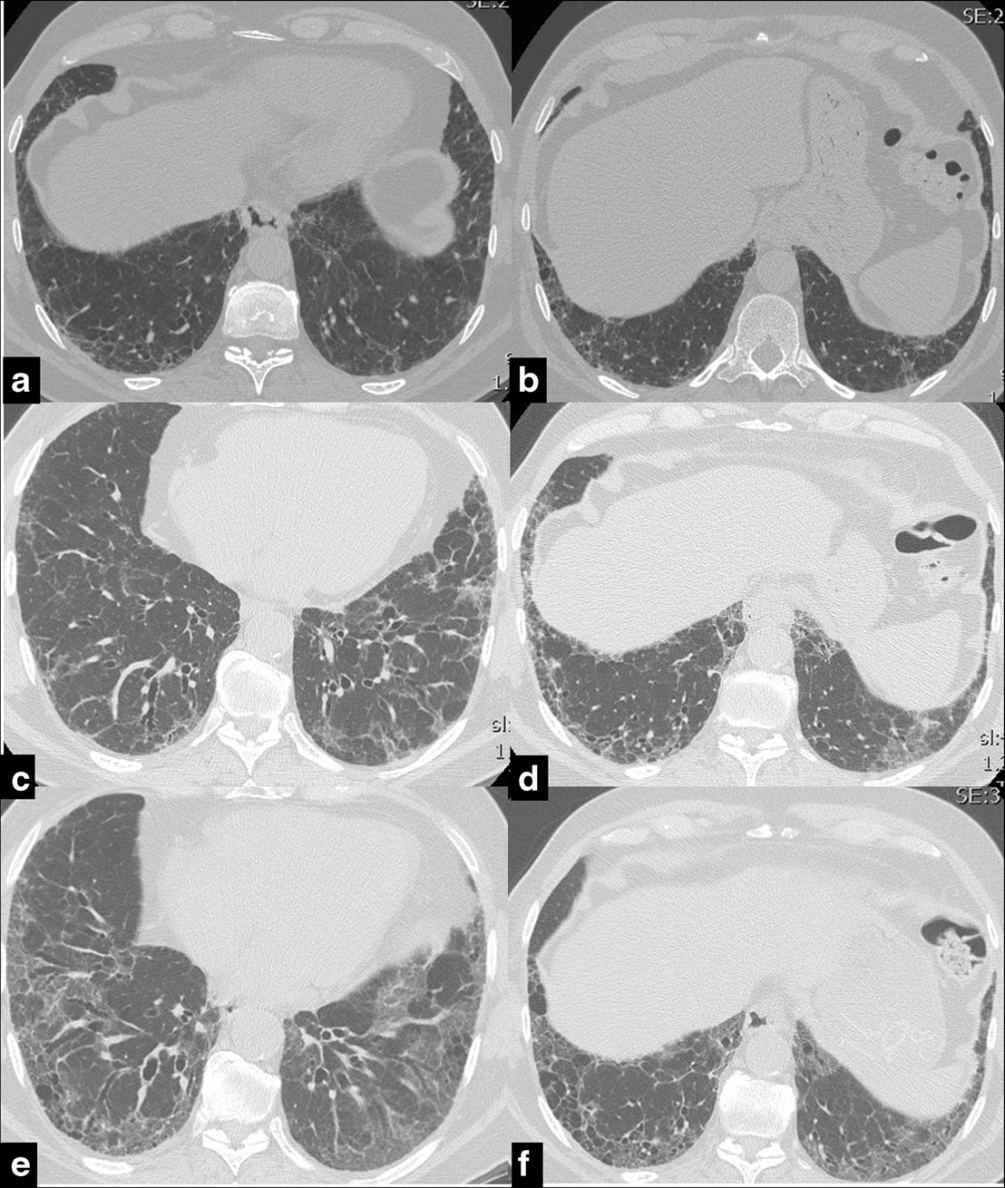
Serial CT images of a 63 years old male affected by IPF. UIP pattern has been diagnosed through surgical lung biopsy at diagnosis. CT shows the progressive worsening of the coarseness. In 2007 (a, b) a moderate peripheral fibrotic reticulation is present. In the following years it progressively gets worse, particularly in the right lower lobe, with increase of the extension of traction bronchiectasis in 2010 (c, d) and with honeycombing and traction bronchiectasis in 2014 (e, f)
